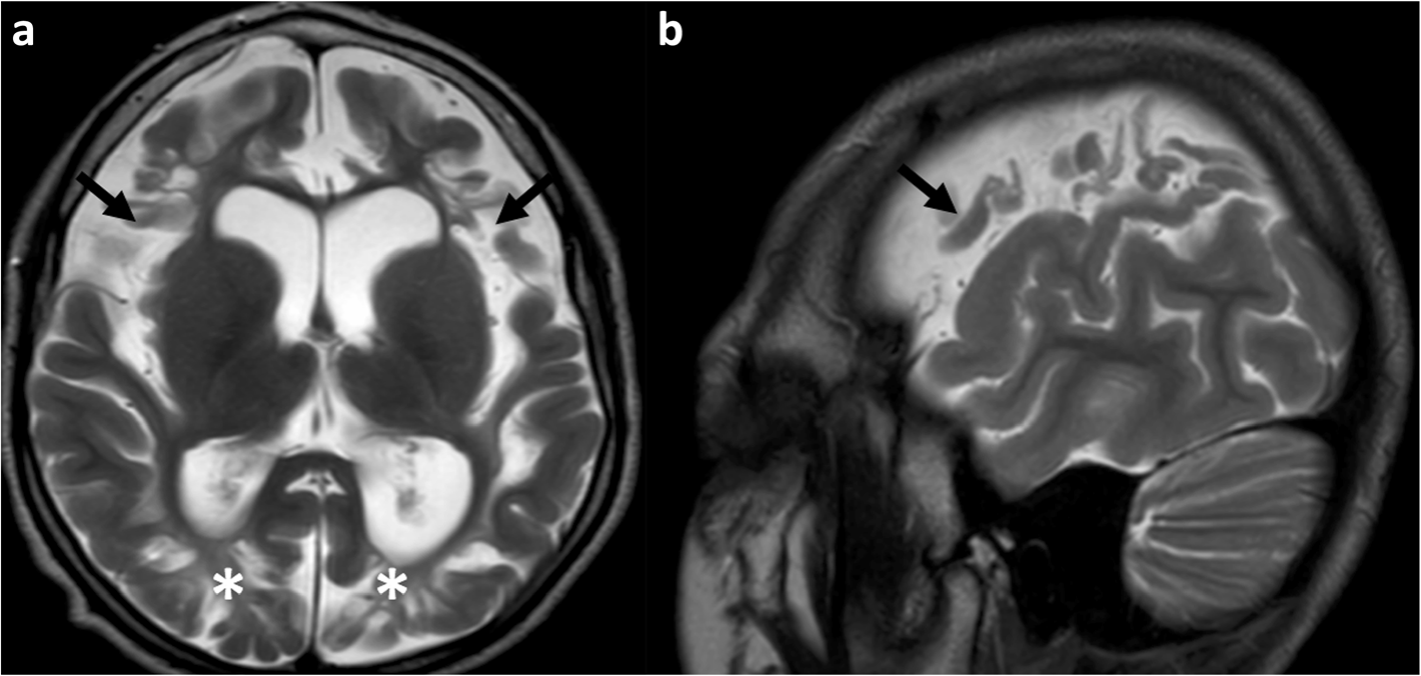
a, b Axial (a) and sagittal (b) T2-weighted MRI in a 6-year-old boy who sustained a partial-prolonged hypoxic-ischaemic injury at term gestation, demonstrates extension of injury from the para-falcine portion of the parasagittal zones, inferiorly anteriorly along the Sylvian fissure (involving the peri-Sylvian zone), with involvement of the inferior aspect of the frontal lobe (arrows) and sparing of the superior temporal lobe. There is also ulegyria in the posterior inter-arterial watershed region (asterisks), visible on the axial T2 image (a)
